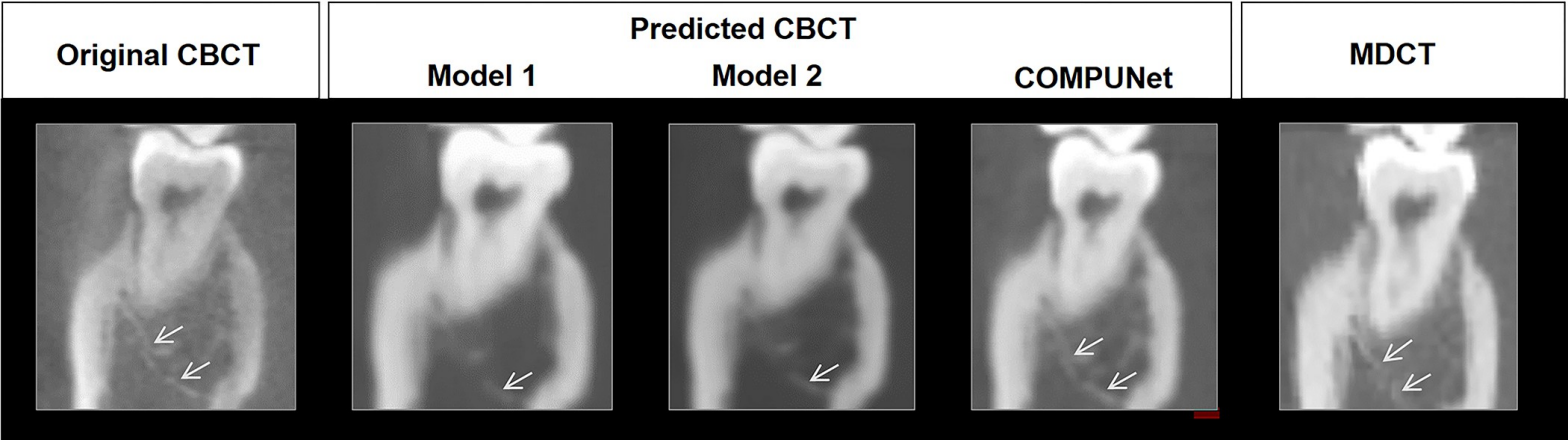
The trabecular bone pattern (arrow) in the same image slides. The fine bone details are best preserved in the predicted CBCT of the proposed model, COMPUNet, compared to that of Model 1 and Model 2.
